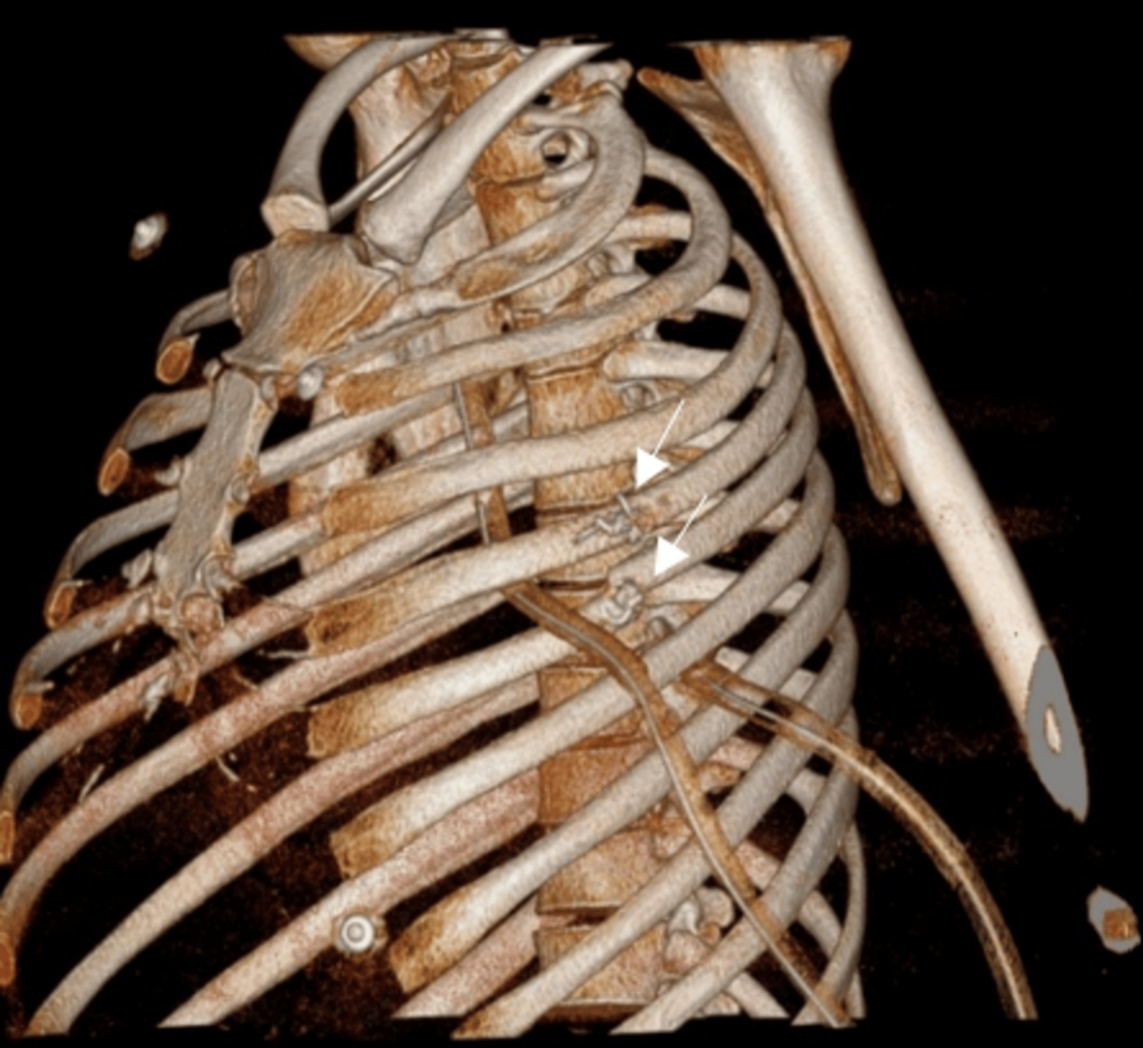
Volume-rendered three-dimensional reconstructed chest CT image shows the wiring of the fourth and fifth ribs (white arrows). ICD tubes are also seen. ICD: implantable cardioverter defibrillator
